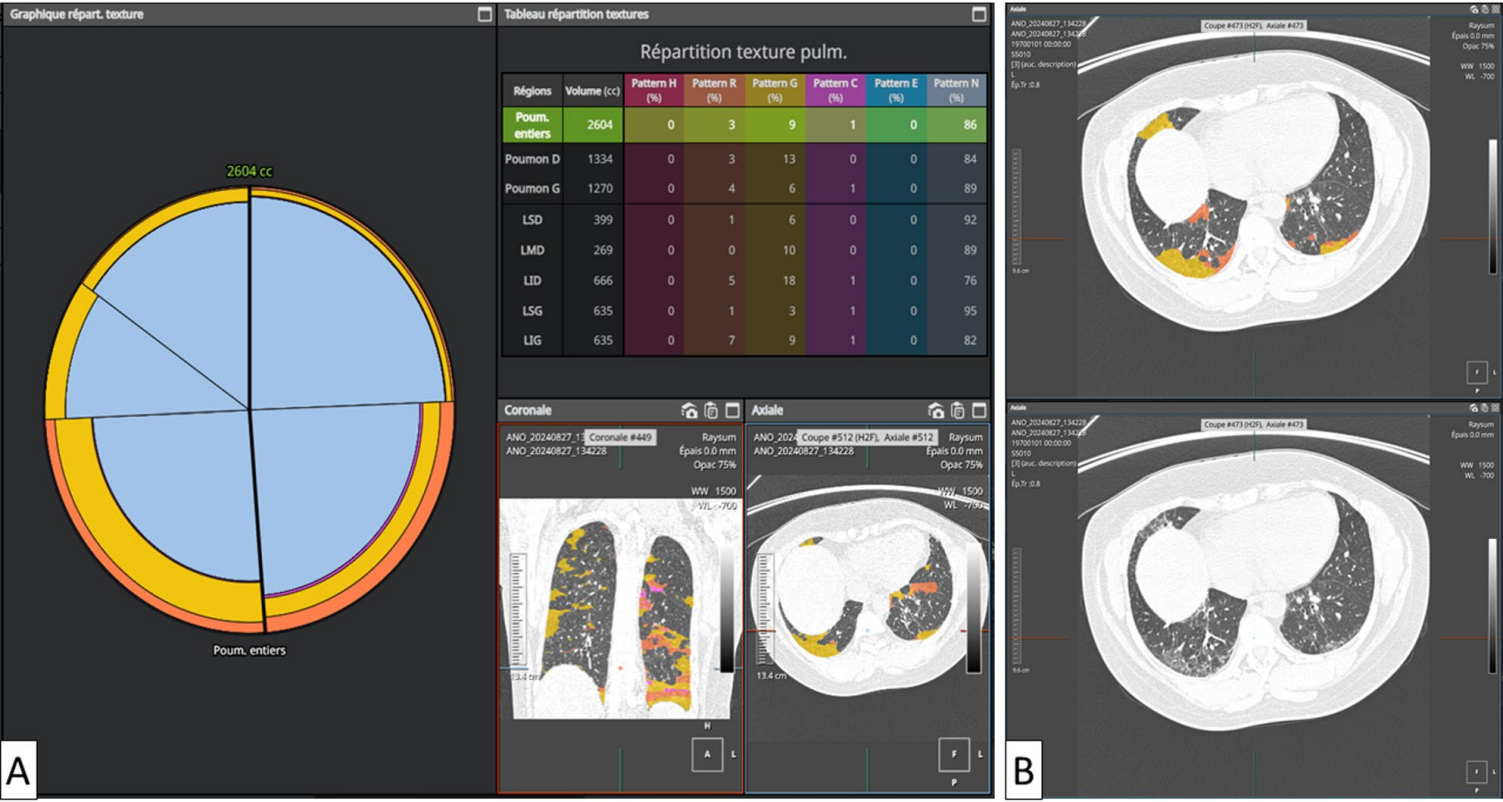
Lung texture analysis in a 47-year-old woman with anti-Jo1–positive idiopathic inflammatory myopathy–associated interstitial lung disease (IIM-ILD). Axial high-resolution CT (HRCT) images are shown at two adjacent basal levels (lower lobes); the patient’s right side is displayed on the left. A Quantitative report summarising the volumetric extent of each texture class, expressed as a percentage of total lung volume and for each lobe. Texture classes are labelled as follows: N (normal lung), G (ground-glass opacities), C (consolidation), R (reticulation), H (honeycombing), and E (emphysema). B Axial HRCT image showing diffuse, predominantly lower-lobe ground-glass opacities with the AI-generated colour-coded texture overlay (top) and the corresponding original HRCT image (bottom), consistent with a predominantly inflammatory, non-fibrotic ILD pattern that may inform immunosuppressive management
